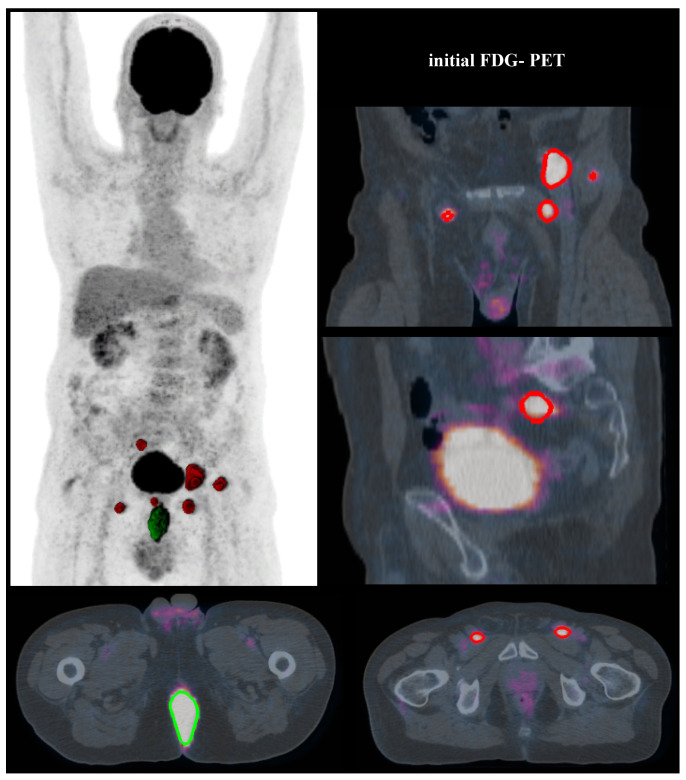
A T4N0 anal SCC was diagnosed at a 71-year-old patient. Initial FDG-PET modified the tumour staging as T4N3 due to an observed extended lymph node involvement (bilateral inguinal, perirectal and external iliac positive nodes). The T-MTV was 70.1 cm^3^ (with 35% SUVmax threshold). Imaging showed residual disease three months after the end of treatment, confirmed by pathological results of surgery. (Segmented volumes: for tumour in green, for lymph nodes in red).
